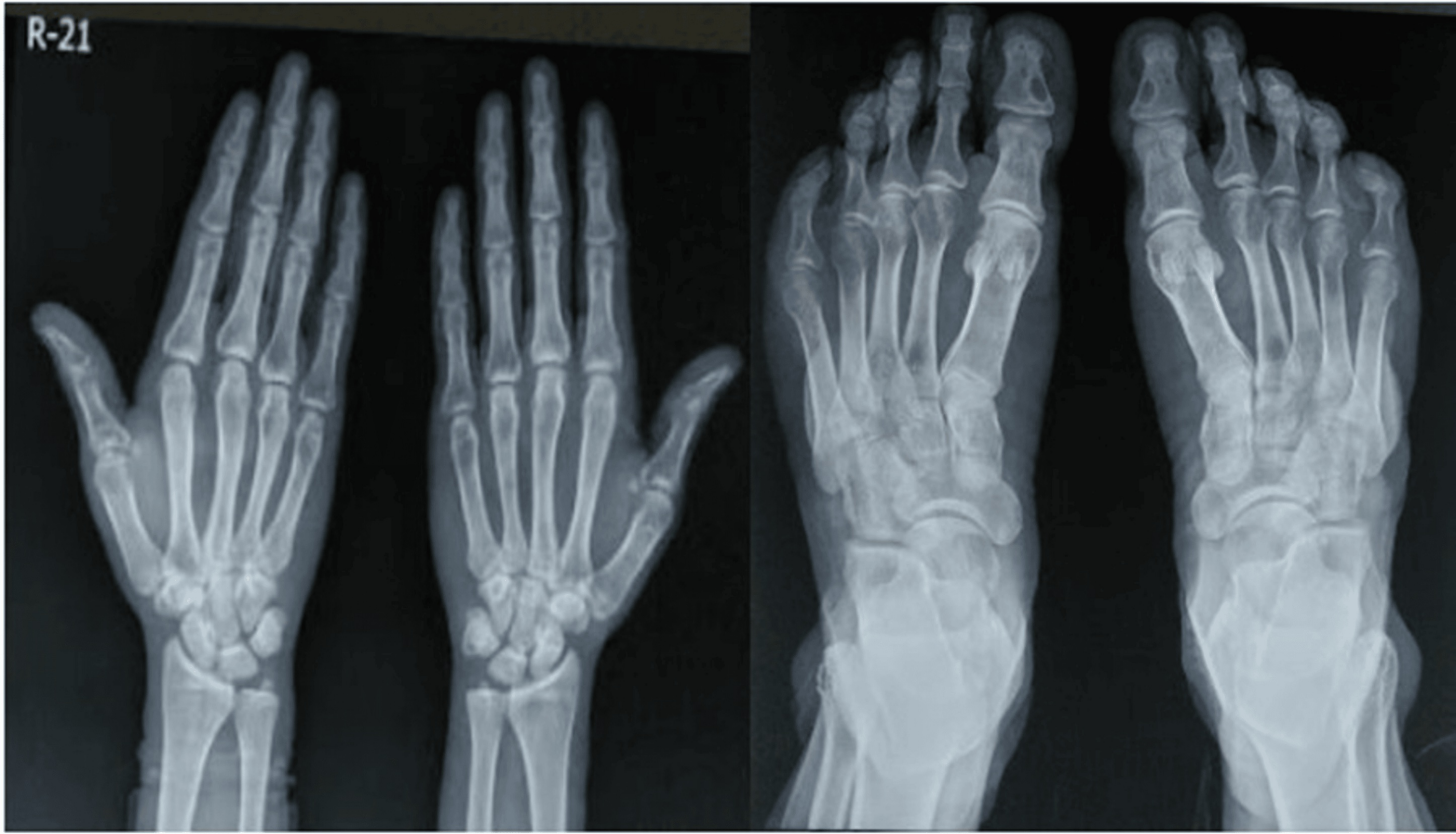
Radiographs of wrists (left) and feet (right) show no significant bony involvement at the sites of the tendon xanthomas.
